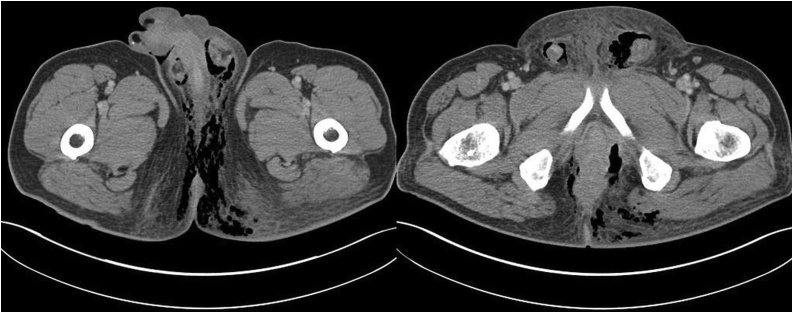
Axial computed tomography image of abdomen and pelvis showing rectal tumor with necrotizing fasciitis in perineal area.
